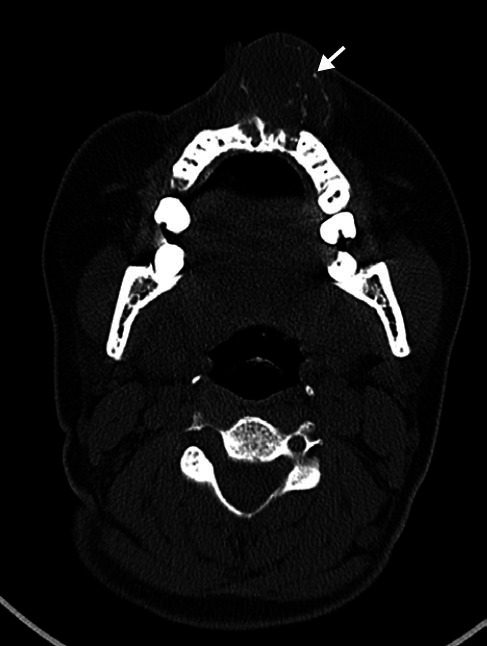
CT scan of an 18-year-old female patient, showing a solid expansile
